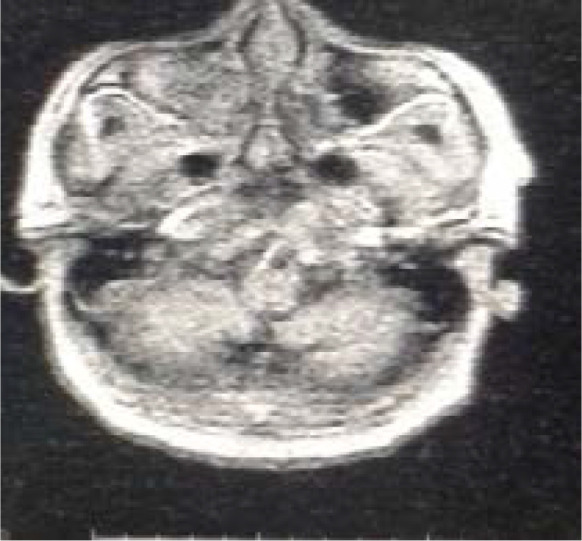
Susceptibility artifact seen on coronal brain MRI.
